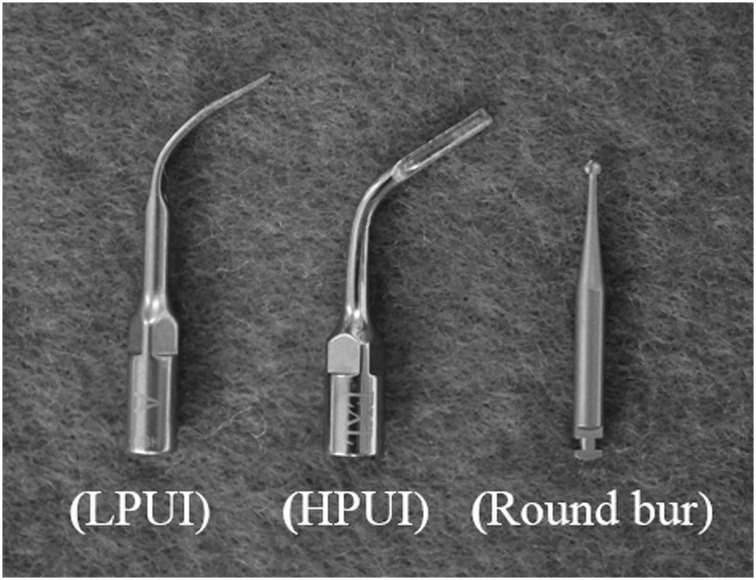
Morphological features of the tips of the ultrasonic instruments and the low‐speed handpiece rotary instrument, consisting of a round steel bur.
